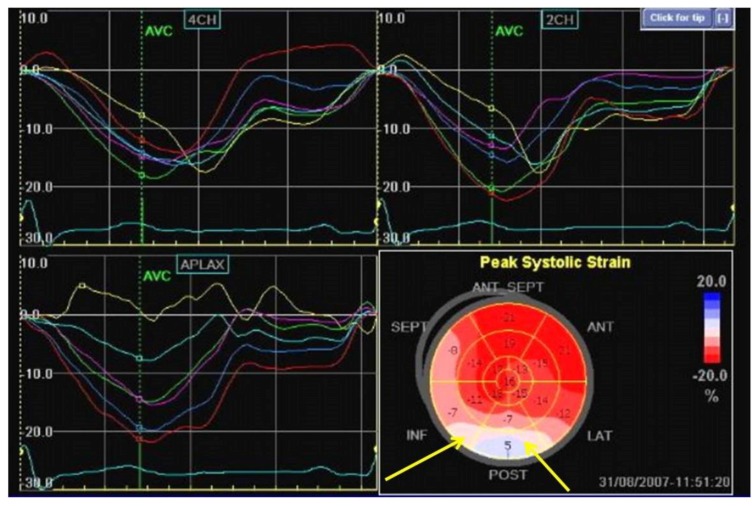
Speckle tracking echocardiography evaluation in patients with previous inferior myocardial infarction notes a reduction of strain value in medium and basal segments of inferior and posterolateral walls (yellow line).
